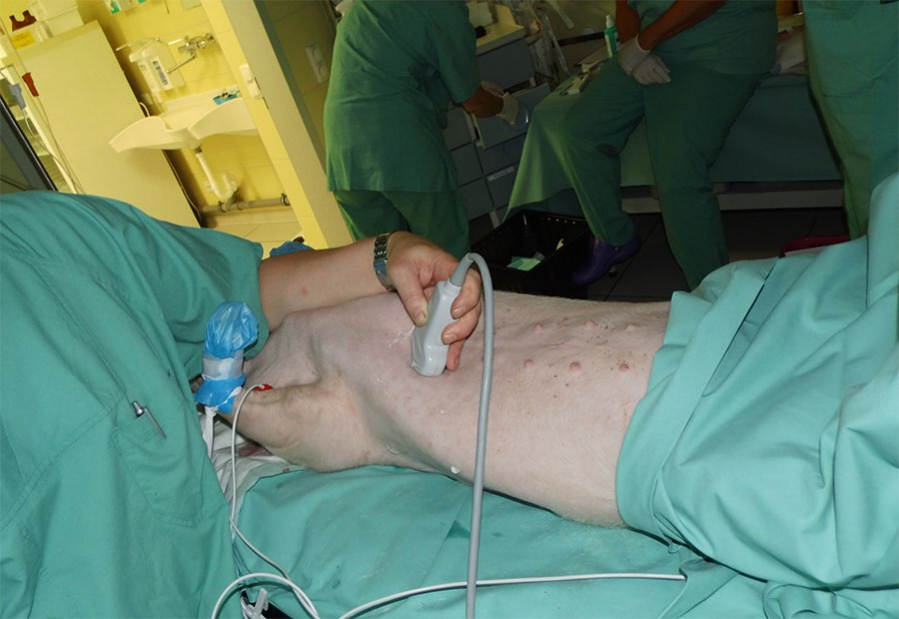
Subcostal approach for the measurement of movement of the right hemidiaphragm by ultrasound (curved array C60e, 5–2 MHz; MikroMaxx; SonoSite, Inc.)
